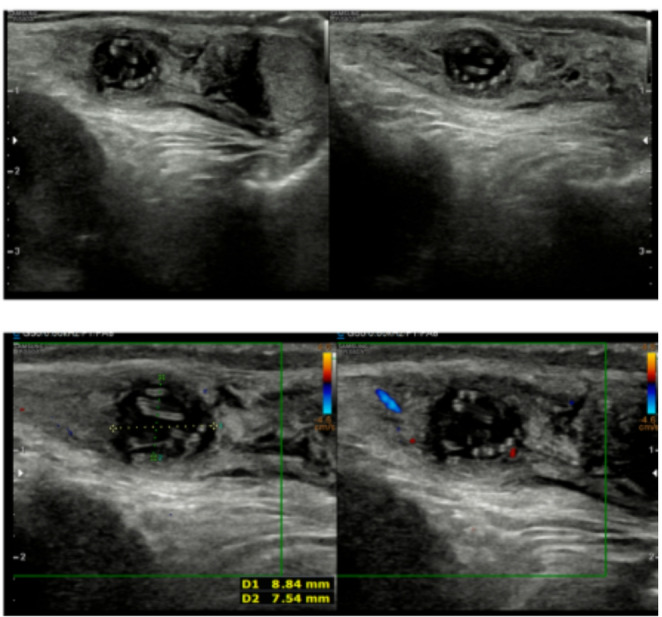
Ultrasound demonstrating a hypoechoic nodule containing linear hyperechogenic lines (worm in-sac-sign)
